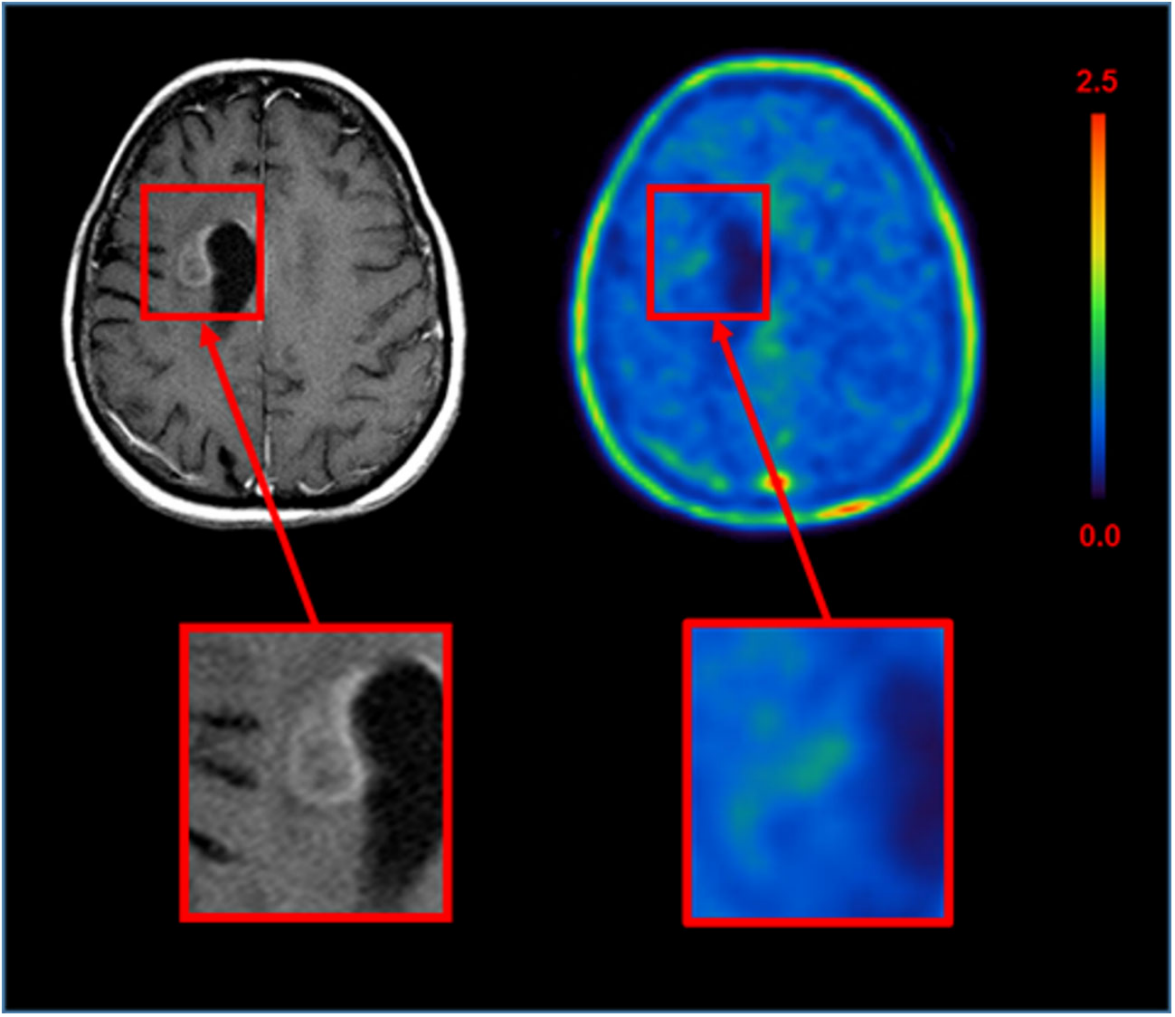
Example of a patient with a glioblastoma multiforme after radiotherapy: on MRI, there is a remaining contrast enhancement reflecting major BBB disruption; in the ^18^F-GE180 PET, no relevant corresponding tracer uptake can be found, showing that a mere macro-disruption of the BBB does not necessarily lead to a highly elevated, nonspecific tracer accumulation (PET image is SUV scaled)
